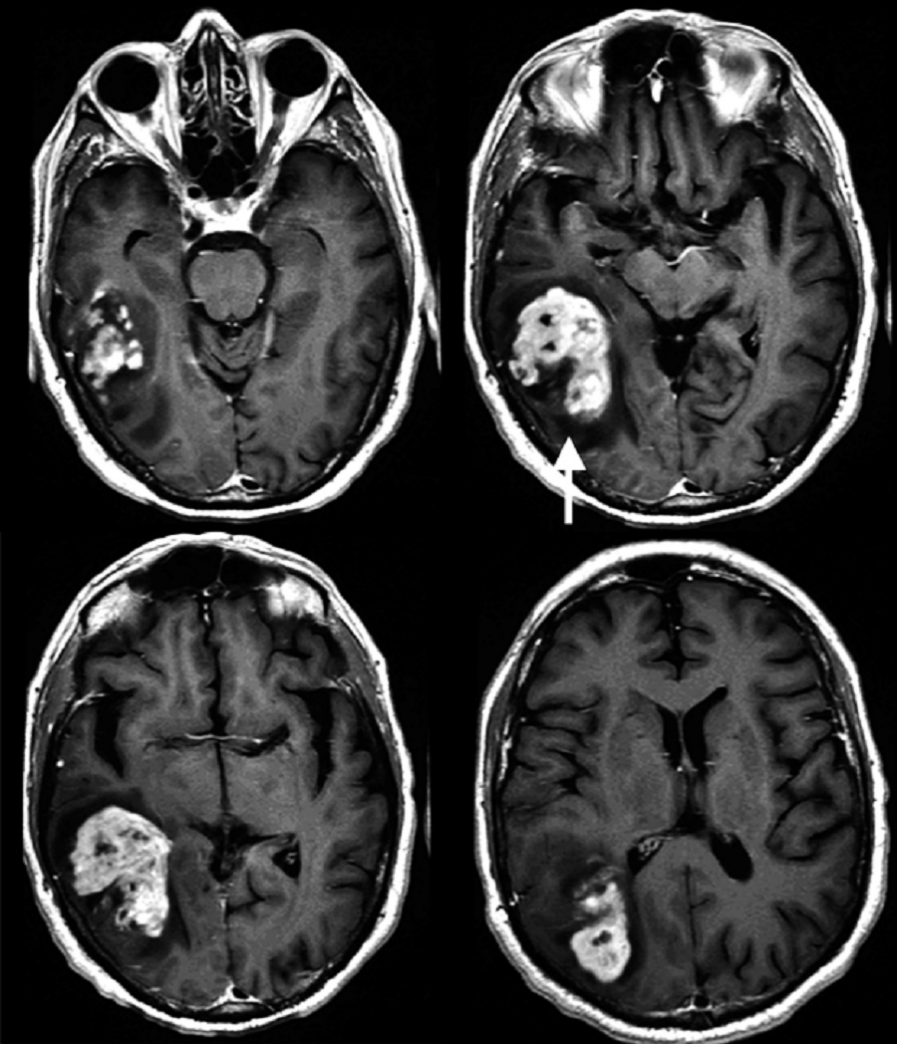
Axial T1 post-contrast MRI Interval progression of heterogeneously enhancing mass now involving the right temporal, parietal, and occipital lobes with local mass effect and surrounding vasogenic edema (arrow) MRI: magnetic resonance imaging
